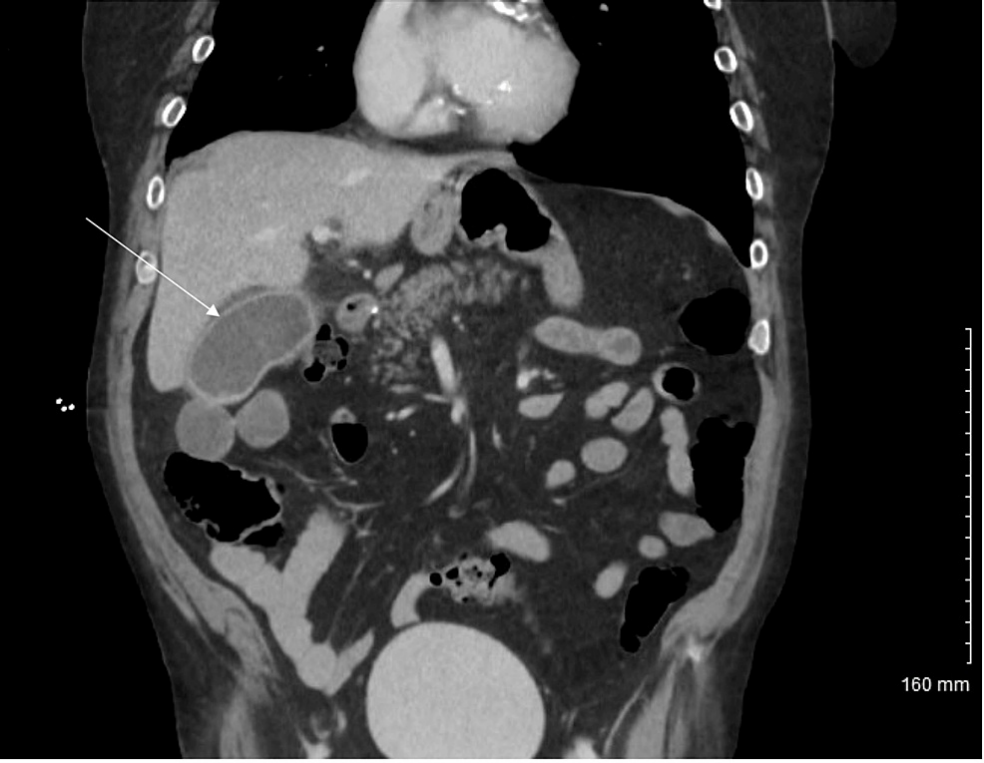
CT of the abdomen and pelvis coronal section showing distended gallbladder with mucosal hyperenhancement and trace pericholecystic fat stranding (white arrow). CT: computed tomography
